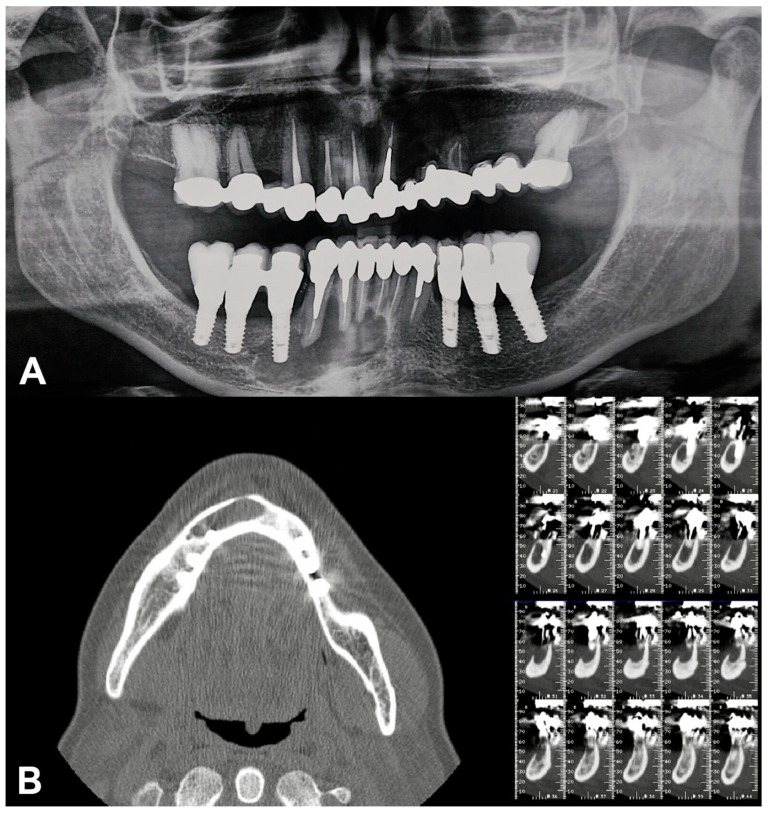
Pre-surgical radiographical examinations. (A) Orthopantomography; (B) Cone Beam Computed Tomography.
